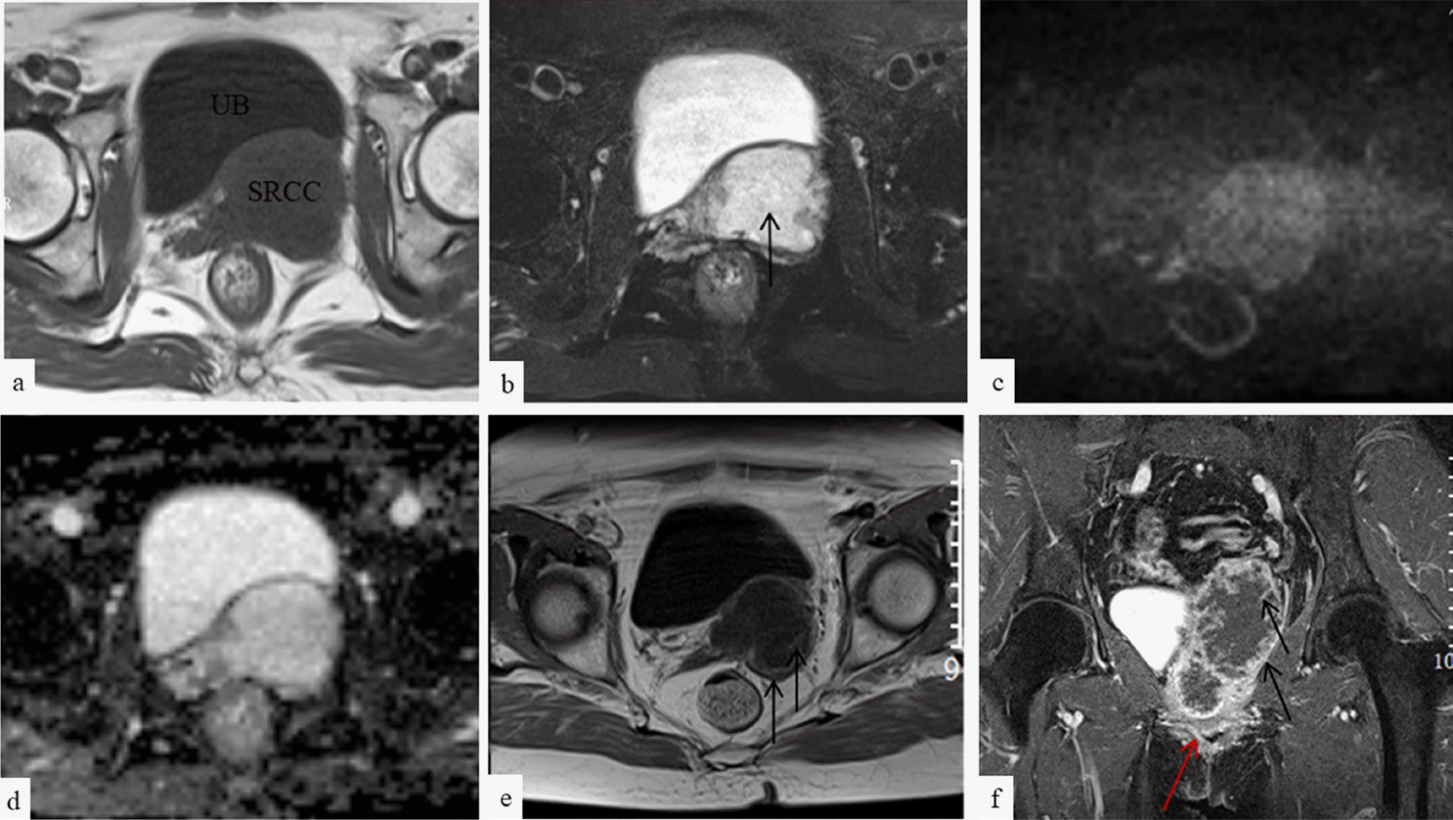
MRI image shows vaginal signet ring cell carcinoma. Axial *T*
